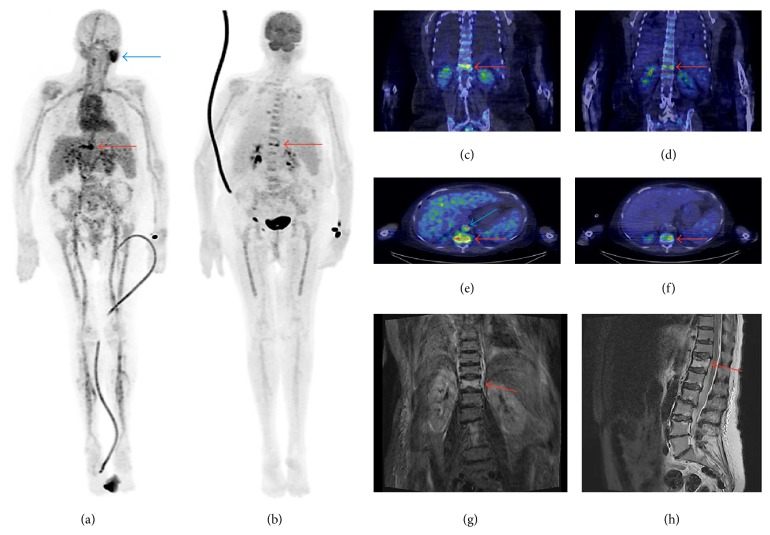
Patient #2 was a 70-year-old woman (weight: 69 kg), with multiple background diseases, who was admitted to hospital because of back pain and high fever. Both ^68^Ga-citrate (a, c, e) and ^18^F-FDG PET/CT (b, d, f) showed vertebral osteomyelitis (spondylodiscitis) in Th12* (red arrows)* and pneumonia in both lungs. MRI showed oedema in Th12 (g, h). ^68^Ga-citrate PET/CT also revealed uptake in the left parotid gland (unspecific; (a),* blue arrow*), neck lymph nodes (reactive), and inferior vena cava (thrombosis; (e),* blue arrow*). There was no ^18^F-FDG uptake in these areas. The injected radioactivity dose of ^18^F-FDG was 279 MBq and the PET acquisition started 50 min after injection. The injected radioactivity dose of ^68^Ga-citrate was 199 MBq and the PET acquisition started 100 min after injection. MRI sequences were as follows: T2-weighted short inversion time inversion recovery (STIR) on the coronal view image (left) and T2-weighted on the sagittal view image (right).
